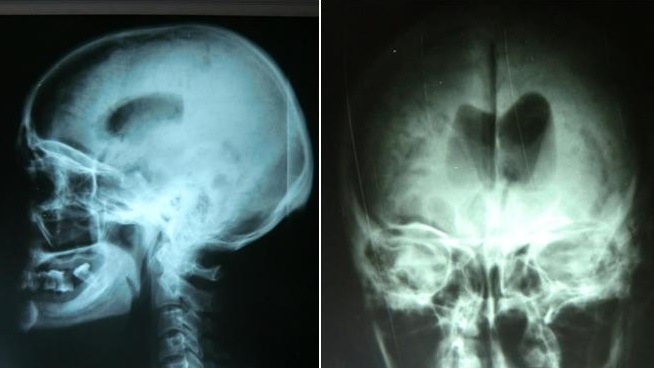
Radiographie du crâne face et profil objectivant une pneumocéphalie avec pneumoventriculie
